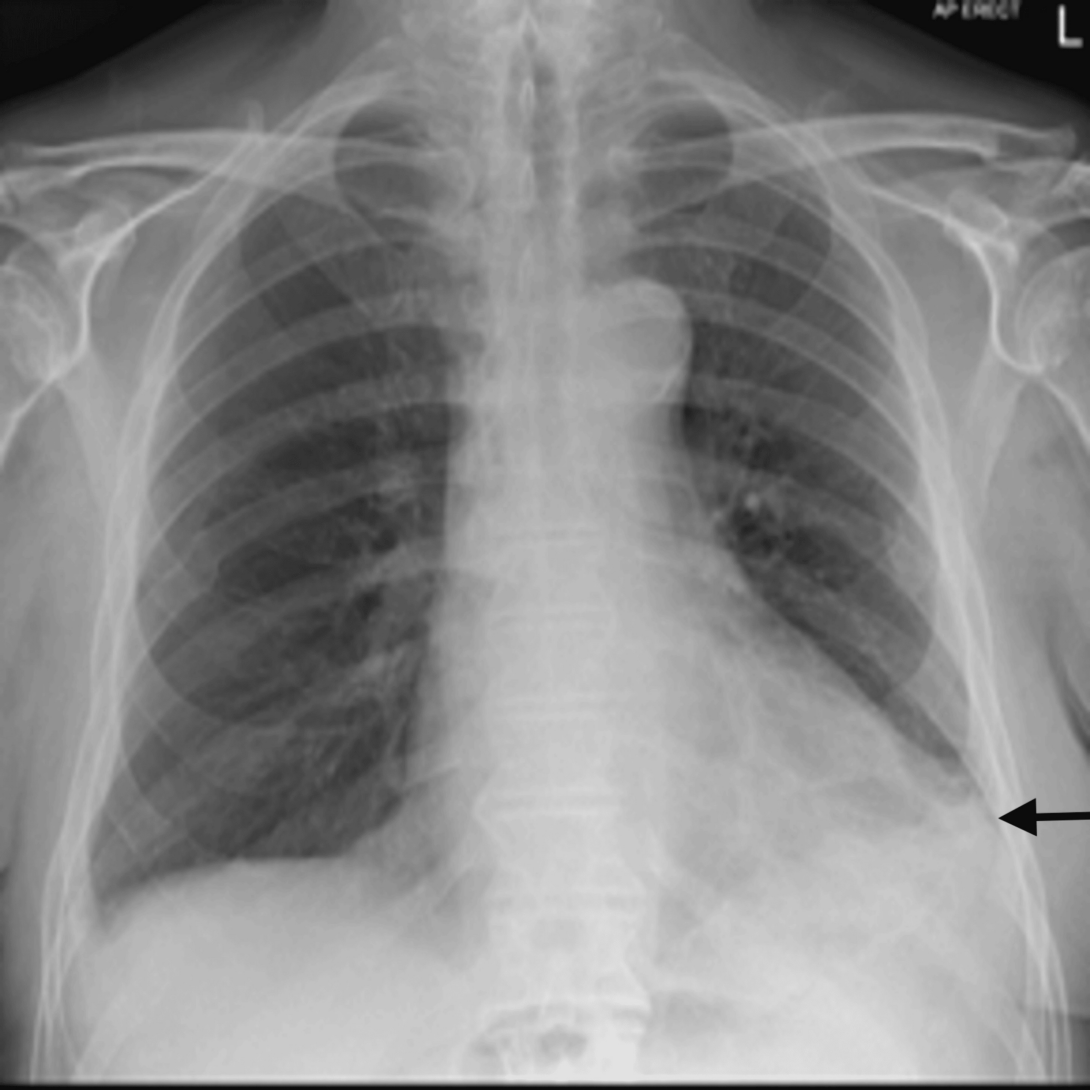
Chest X-ray: small left pleural effusion with left basal consolidation. This is an initial Chest X-ray at the time of admission to the hospital.
